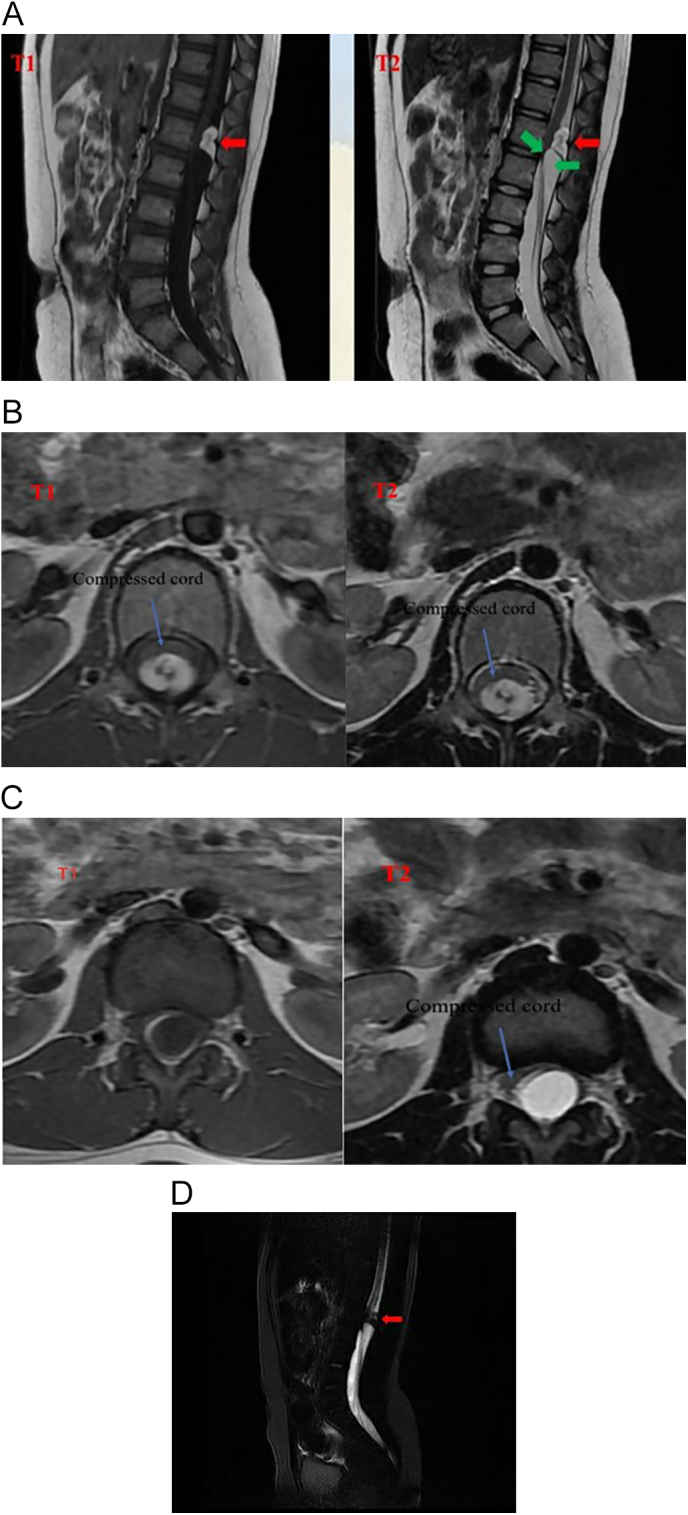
A, Preoperative sagittal T1-weighted MRI shows two lesions with different signals. The upper lesion was hyperintense, extending along the first lumbar vertebra on both T1 and T2 images corresponding to the characteristics of an intradural lipoma. The inferior lesion was hypointense on T1 image and hyperintense on T2 image indicating an intradural cyst. B, Axial MRI shows hyperintense lesion on both T1 and T2 images. C, Axial MRI shows a lesion that is hypointense on T1 and hyperintense on T2. D, T1-weighted MR image with fat suppression demonstrates the absence of the lesion.
